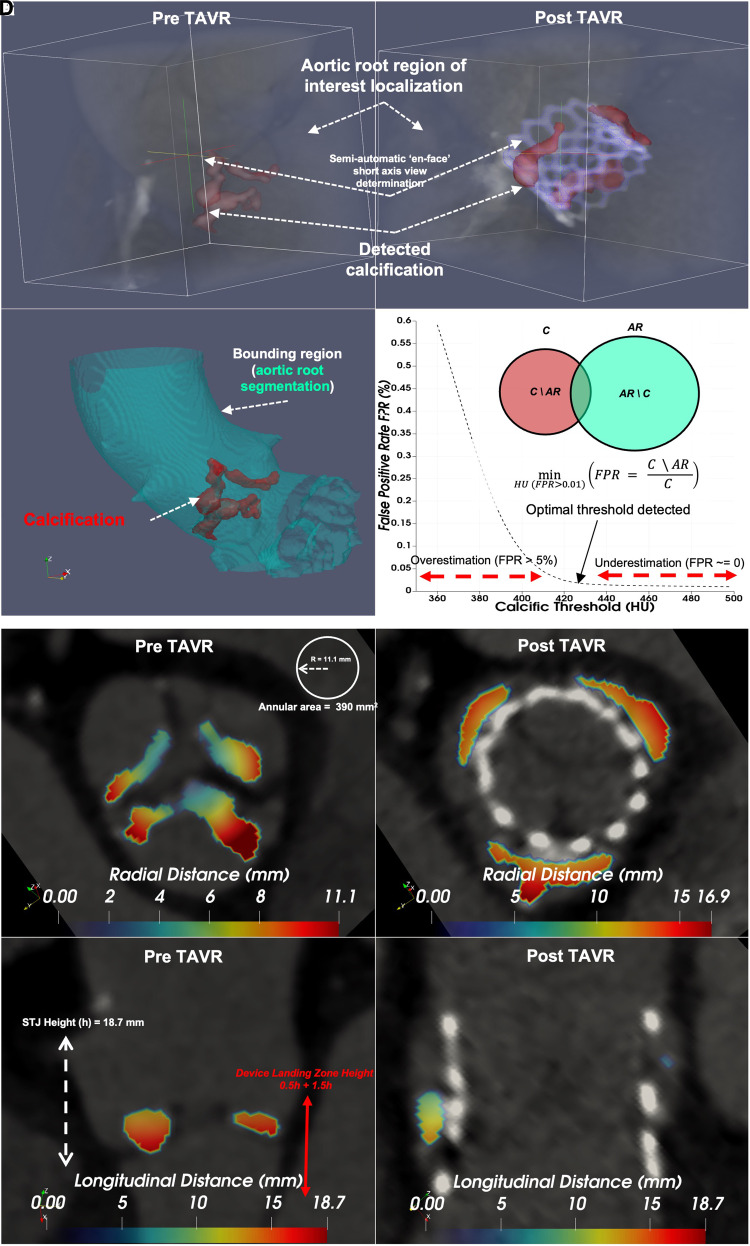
Visual representation of the computational framework developed for the assessment of calcification pattern and structure in contrast enhanced CT images for TAVR; (*A* and *B*) detected calcific segments are overlaid on the aortic root segmentation and device stent (outlined) before and after TAVR; (*C* and *D*) the FPR method detects calcification by optimizing the false positive rate between aortic root lumen and calcific segments (outlined); (*E* and *F*) the 2D ‘en-face’ short axial view near the annular plane shows calcification distances to the annular centre in a range from 0 to the annular radius; (*G* and *H*) the 2D long-axis view before and after TAVR shows calcification distances to the annular plane in a range from 0 to the height of the sinotubular junction (STJ).
